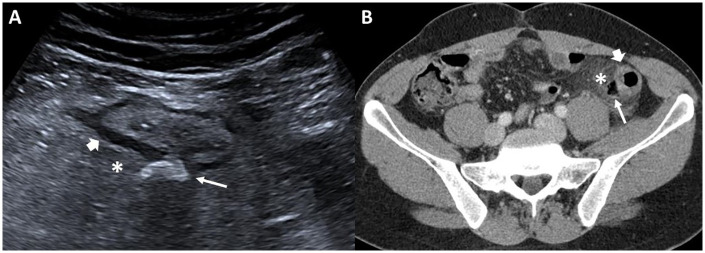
Acute left colonic diverticulitis stage 1a. A US image obtained with a convex transducer in an oblique plane (A) shows a thickening of the left colon (short arrow), with hyperechoic fat (*) with echogenic foci adjacent to the wall of the colon (large arrow). A contrast-enhanced CT in the axial plane (B) demonstrates similar findings: thickening of the left colonic wall (short arrow) with pericolic inflammation (*) and a bubble of gas (short arrow).
